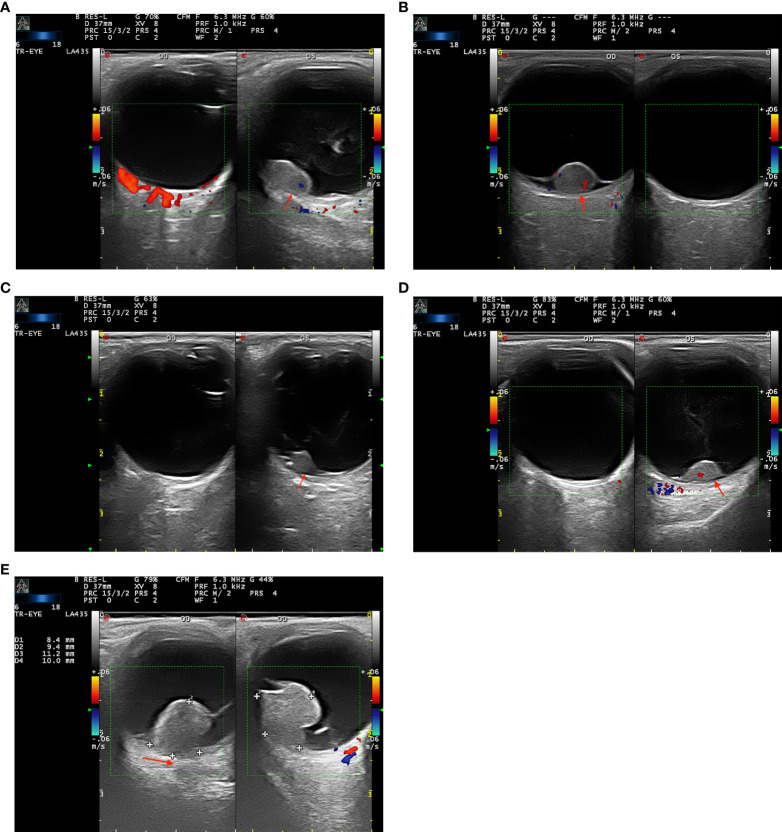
(A–D) Color Doppler imaging (CDI) shows the “black-linear” sign in three patients with retinal pigment epithelium (RPE) adenoma (A–C) and one patient with (RPE) adenocarcinoma. The well-defined lenticular, oval-shaped masses manifest moderate signal intensity with no choroidal excavation and bulging posterior choroid. The lesions show mild or moderate enhancement and present with the “black-linear” sign (a low signal linear shadow between the lesion and the marked enhanced choroid, red arrow). (E) A 57-year-old woman with uveal melanoma (UM) in the right eye. A mushroom-shaped mass shows a moderate signal intensity with choroidal excavation and bulging posterior choroid sign (red arrow).
